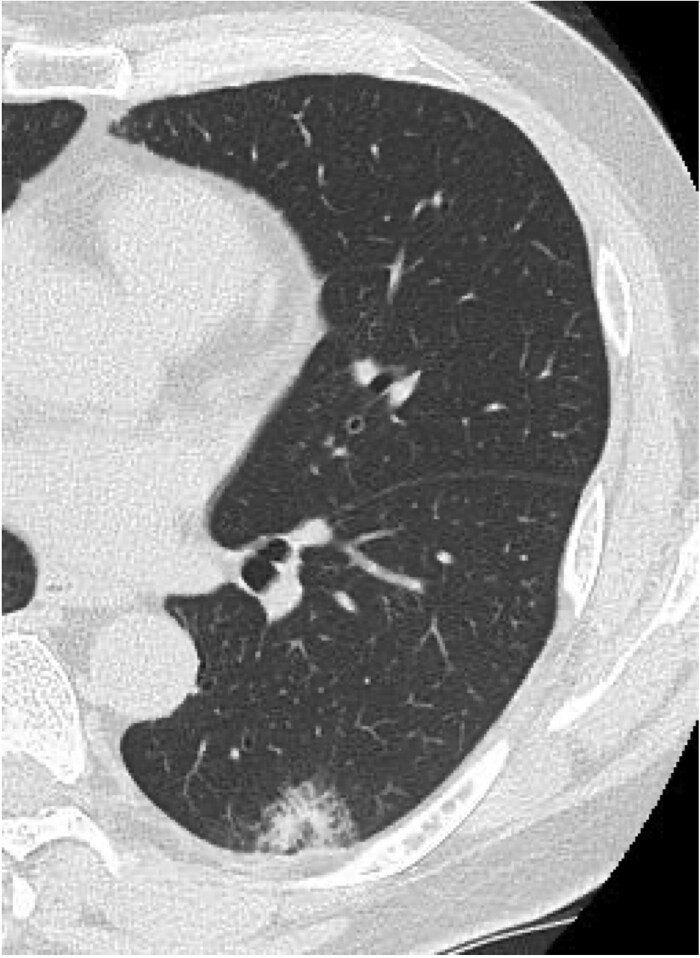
Preoperative chest computed tomography showing a 35 × 12 × 20 mm nodule with mild ground-glass opacity in the left lower lobe (S10).
